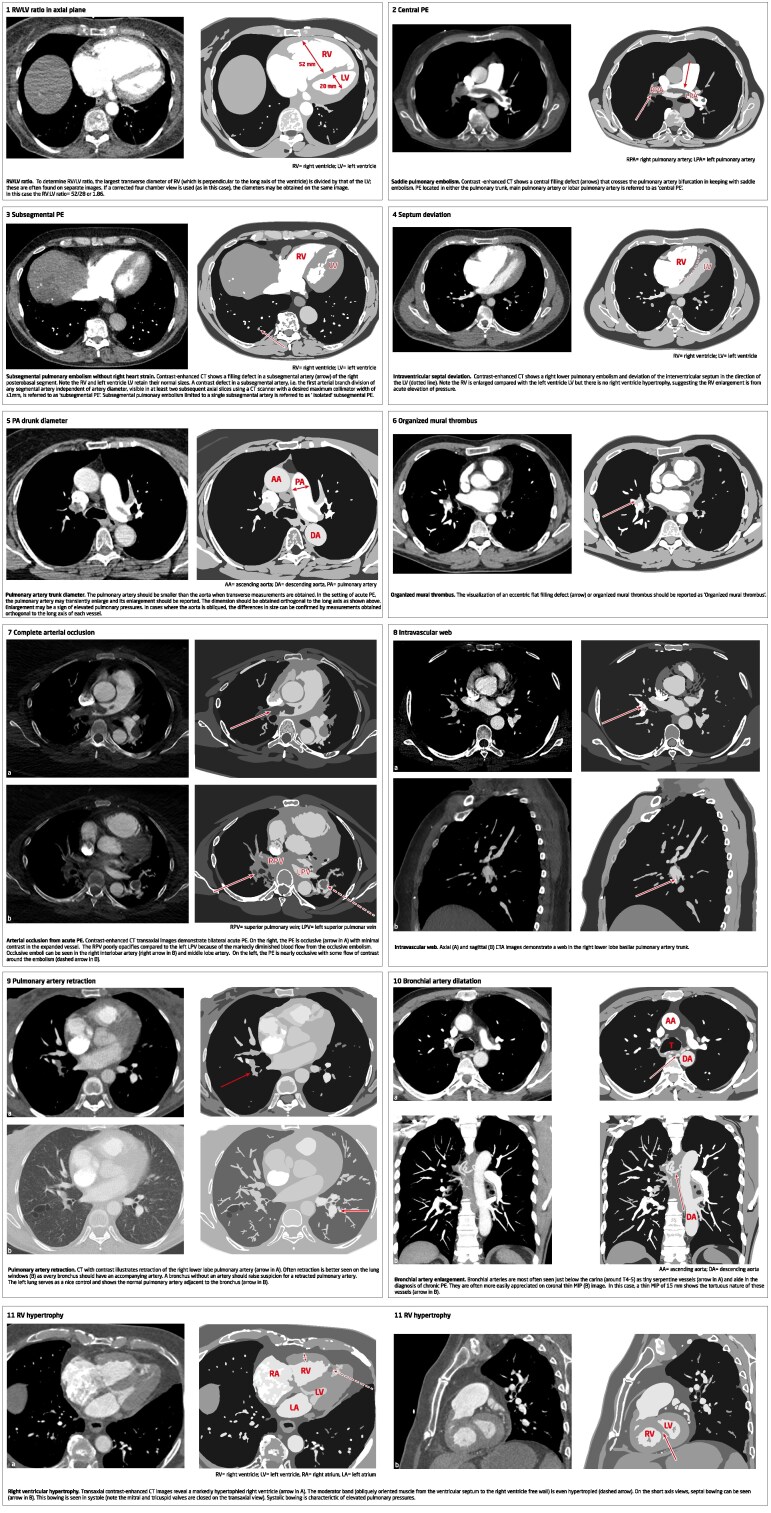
Image atlas of core set of CTA findings to be routinely mentioned in CTA reports.
